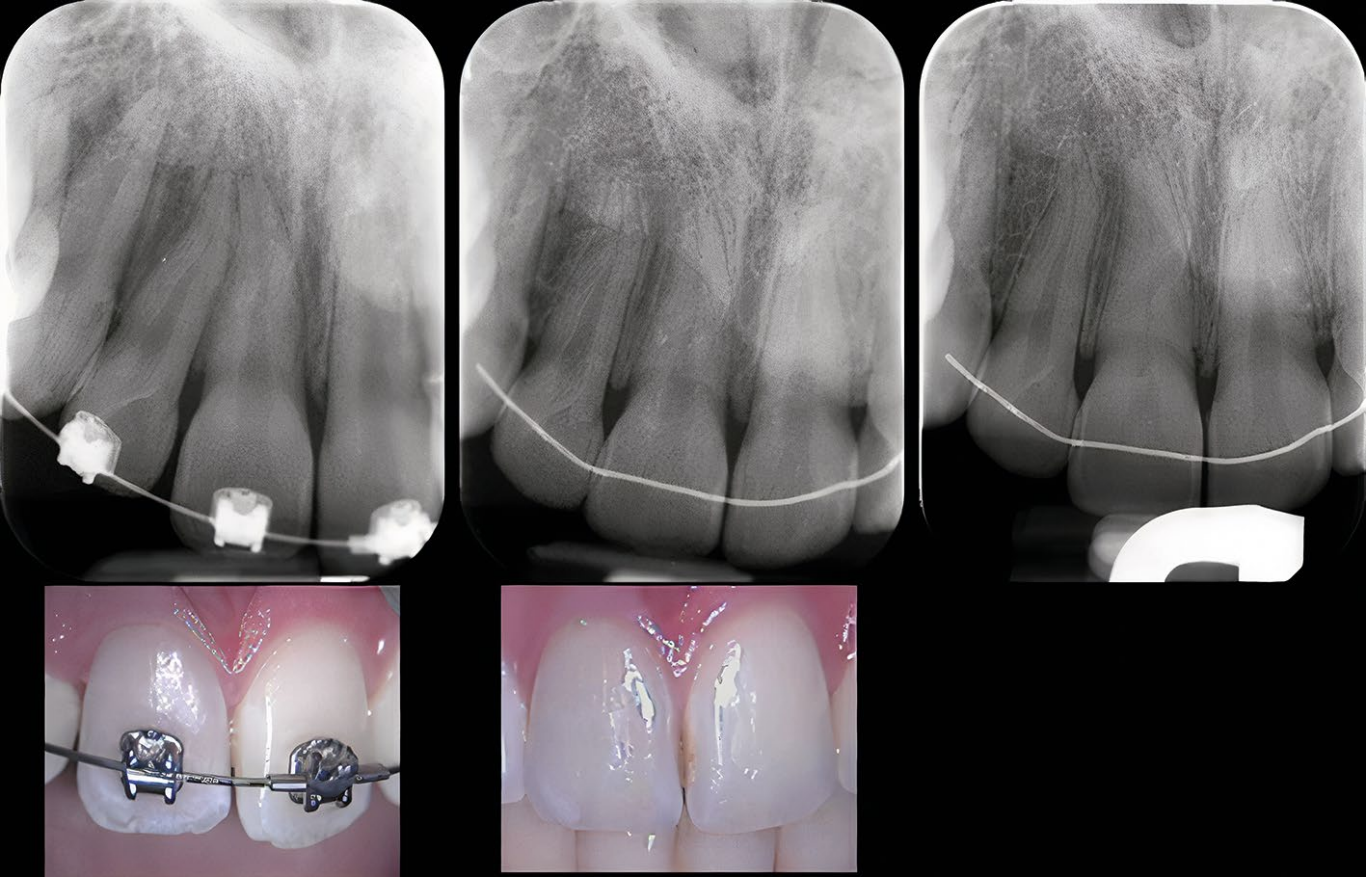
The maxillary right central incisor discoloured during OTM but the pulp was normally responsive to CO_2_ sensibility testing; subsequent reviews showed PCC and improvement in colour. Periapical radiographs at presentation (top left), five-month review (top centre), and 4.3 years (top right). Photographs on presentation (bottom left) and at five-month review (bottom right). Images courtesy of Dr Vijay John
